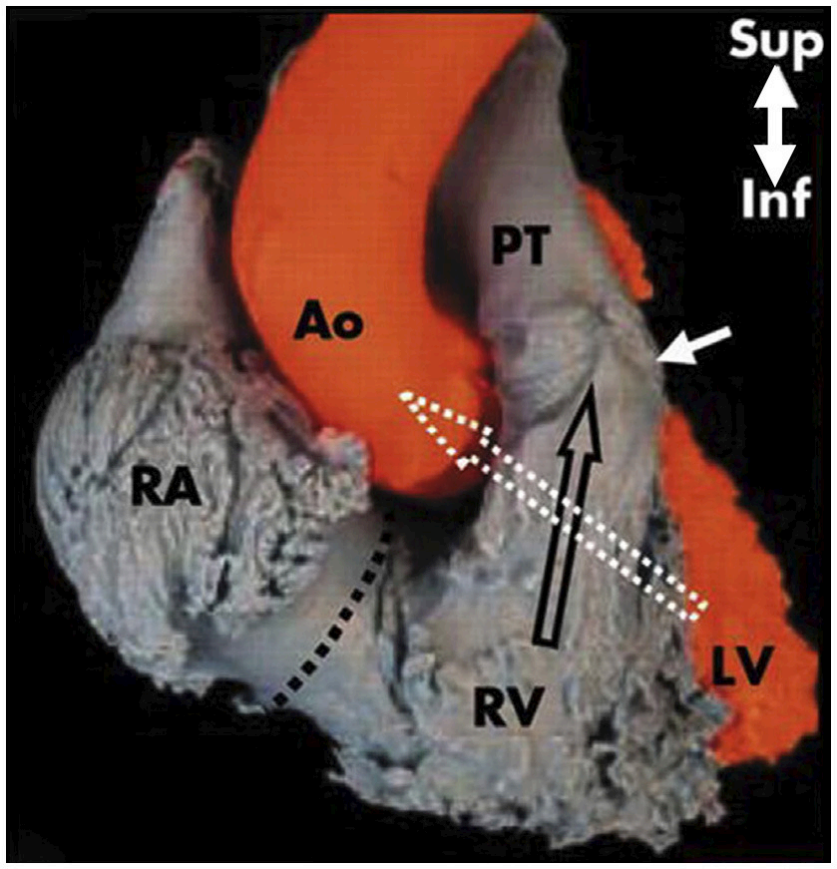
The right outlet portion of the ventricular septum is smooth and has three components. The largest is the infundibular septum, which separates the pulmonary valve (with arrow) from the aortic and TV. A second part of the outlet portion of the septum is the anterior extension of the trabecular septomarginalis (septal band). A third small, very anterior portion is a narrow extension superior to the trabecular septum. The axes of the right and left ventricular outflow tracts differ significantly. That of the RV is almost vertically oriented, whereas that of the left ventricle angles sharply to the right, a characteristic criss-cross feature (white and black empty arrows), visible under fluoroscopy in the left anterior oblique projection and in the parasternal long axis view by two-dimensional echocardiography. RA, right atrium; Ao, Aorta; RV, right ventricle; PT, pulmonary trunk; LV, left ventricle. Modified from Ho et al. (12).
